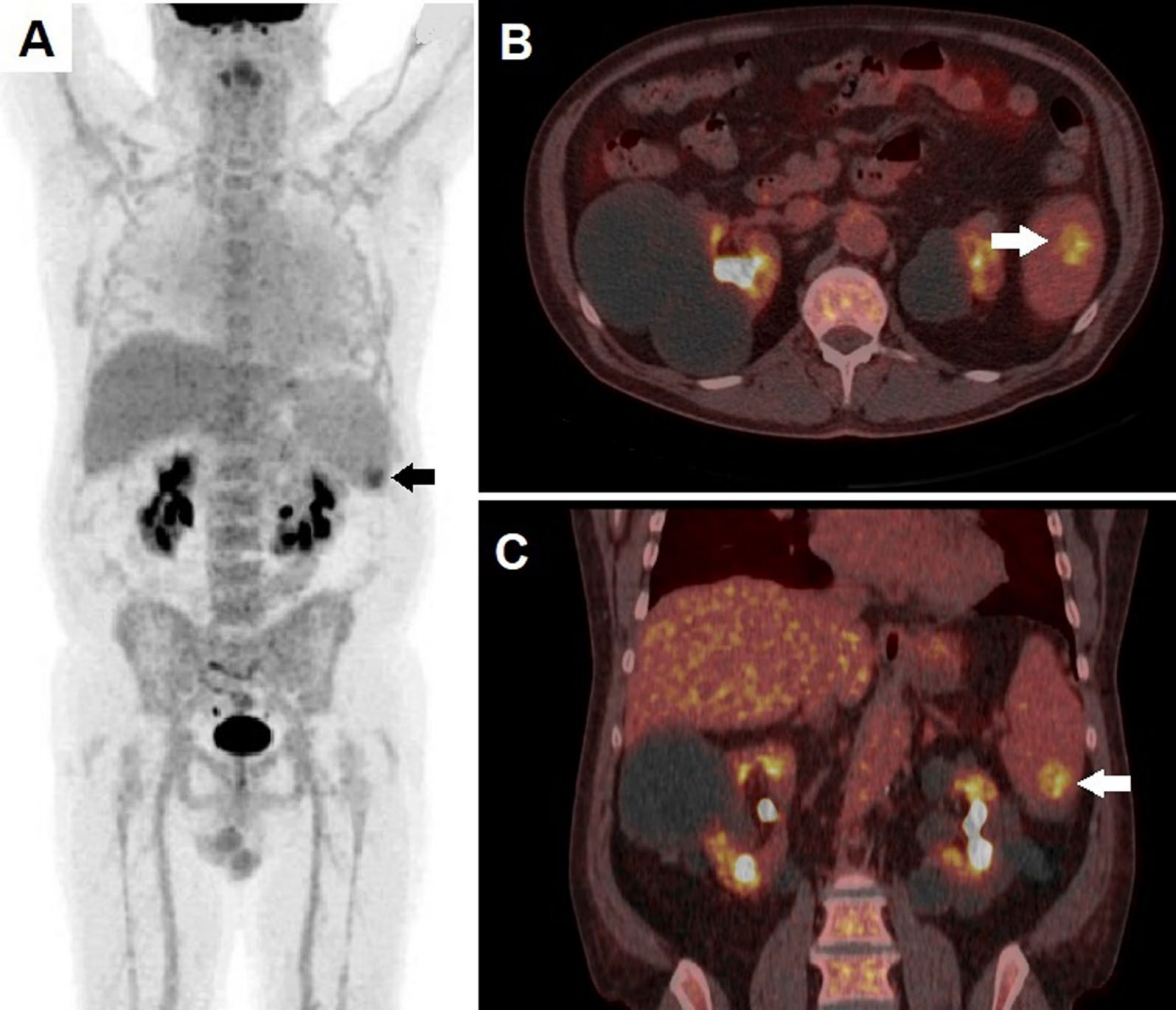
^18^F-FDG PET/CT Whole Body (a), performed at patient's admission, demonstrated an area of increased tracer incorporation (SUVmax 6.1) in the spleen inferior pole (black arrow), associated with diffuse bone marrow uptake. Fused axial (b) and coronal (c) PET/CT slices well depicted focal ^18^F-FDG accumulation (white arrow) within a hypodense area in the inferior splenic pole, with no evidence of meaningful spleen enlargement (splenic length 11 cm)
